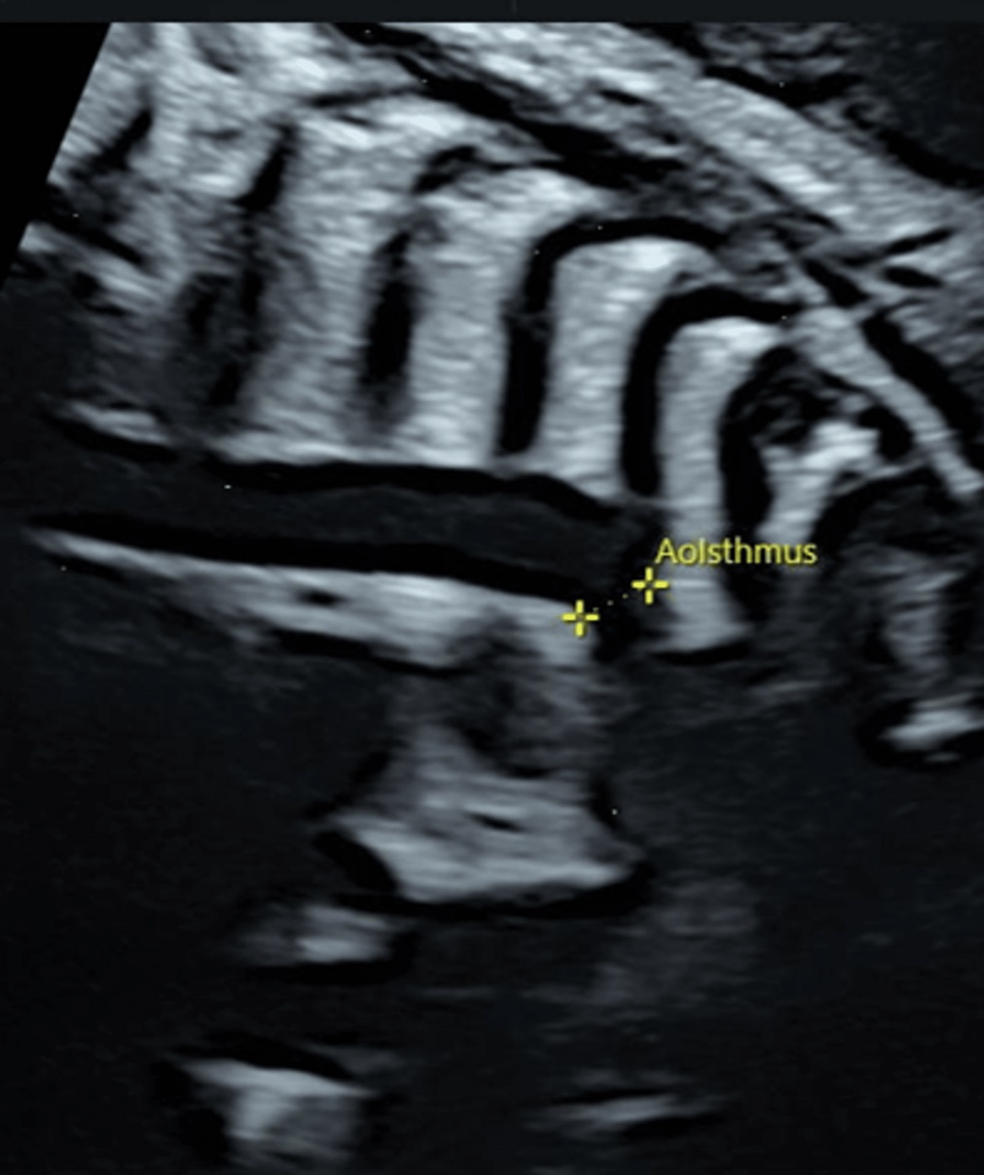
Fetal echocardiography Fetal echocardiography at 35 weeks' gestation revealed aortic isthmus measured in 3.8 mm (z = -2.4).
